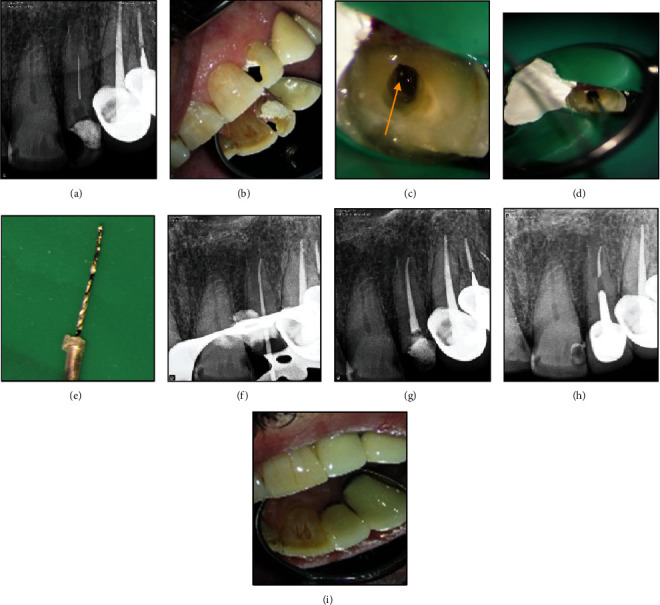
(a) Preoperative radiograph showing a broken instrument in the palatal canal (arrowhead). (b). Intraoral view. (c) Coronal portion of the broken file in the palatal canal was visualized under a DOM. (arrowhead). (d, e) Broken instrument was removed from the canal using the tube-and-glue technique. (f, g) Canal preparation and obturation. (h, i) One-year follow-up: tooth was restored with post and core and metal-ceramic crown.
